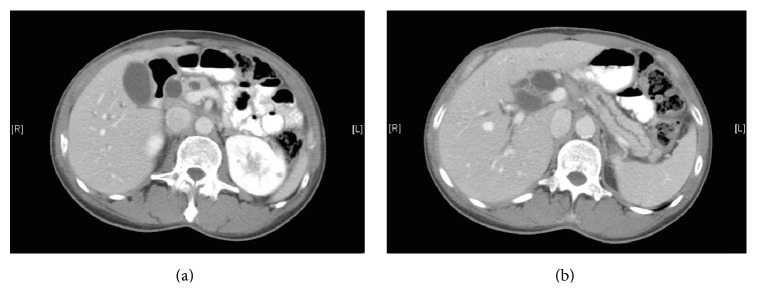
(a) CT abdomen with contrast demonstrating intra- and extrahepatic biliary ductal dilatation. (b) CT demonstrating obvious pancreatic ductal dilatation without identification of discrete mass.
